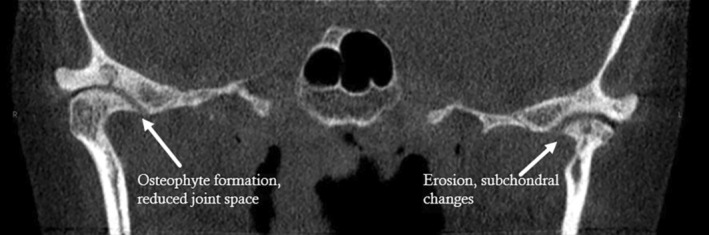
Coronal CBCT view of TMJ with degenerative changes. Coronal view of the TMJ displaying osteophyte formation, reduced joint space and subchondral changes in the articular eminence and right condyle. The left TMJ exhibits cortical erosion and subchondral changes.
